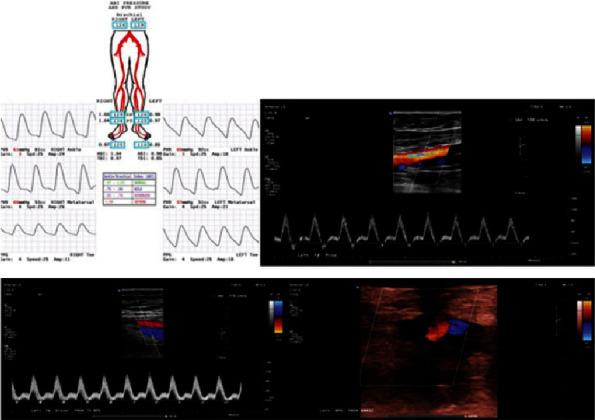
Color Doppler ultrasound/ABI obtained 2 months status postoperative intervention indicating graft patency in both longitudinal and transverse planes.
